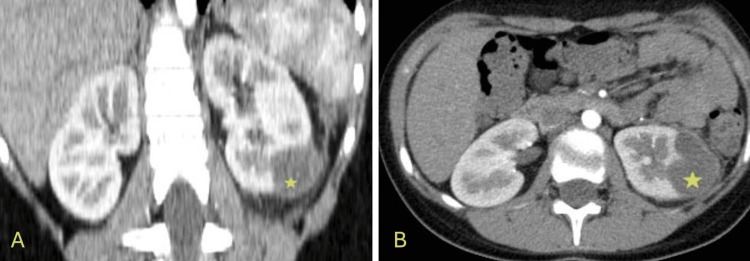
CT scan showing a 3.8cm lower pole left renal collection that is compatible with a renal abscess (yellow stars). A: Coronal view of the CT scan; B: Axial view of the CT scan
